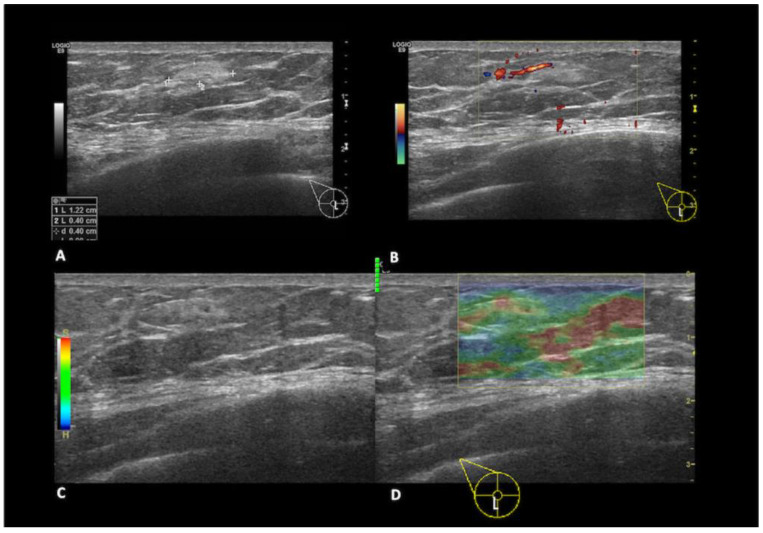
A 49year-old patient with a history of a blunt trauma on the right breast. In the sub-areolar region (A) of the right breast, there is a hyperechoic mass with anechoic internal components and (B) no signs of vascularization at color-doppler. (C,D) Strain elastography evaluation shows that the mass has an intermediate elasticity. The findings are consistent with fat necrosis.
